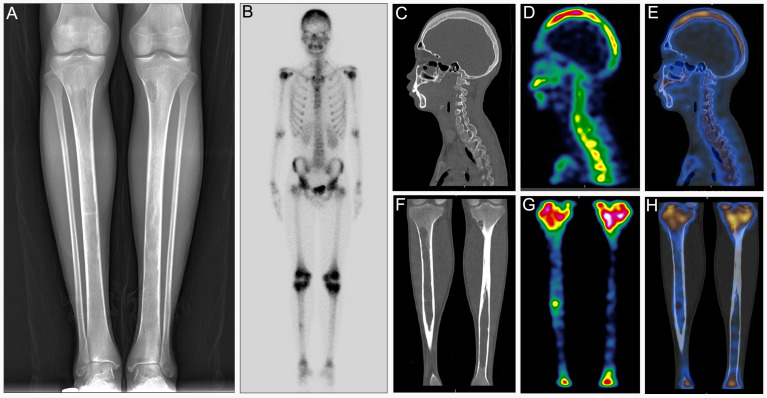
Patient 1: (A) Frontal radiograph of left tibiae demonstrated a well-demarcated lytic lesion that did not show sclerotic borders, endosteal erosion or associated expansion of the tibia shaft. (B) ${}^{99m}$Tc-MDP bone scan showed abnormal concentration of tracer around the knee joints, in the kull and bilateral humeral heads. (C–E) CT, SPECT and fusion images of the head. (F–H) CT, SPECT and fusion images of the both tibiae.
